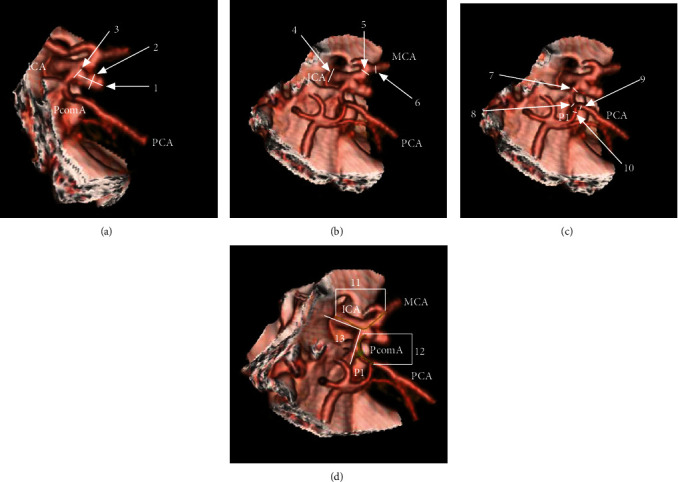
CTA-based measurement parameters are as follows: (a) No. 1 is the height of the aneurysm, No. 2 is the width of the aneurysm, and No. 3 is the width of the aneurysmal neck; (b) No. 4 is the diameter of the intradural ICA at its origin, No. 5 is the diameter of the ICA at its termination, and No. 6 is the diameter of the MCA at its origin; (c) No. 7 is the diameter of the PcomA at its origin, No. 8 is the diameter of the PcomA at its termination, No. 9 is the diameter of P2 at its origin, and No. 10 is the diameter of P1 at its termination; (d) No. 11 is the length of the intradural ICA, No. 12 is the length of the PcomA, and No. 13 is the angle between the ICA and the PcomA. Abbreviations: CTA: computed tomography angiography; ICA: internal carotid artery; MCA: middle cerebral artery; PCA: posterior cerebral artery; PcomA: posterior communicating artery; P1: first segment of PCA.
